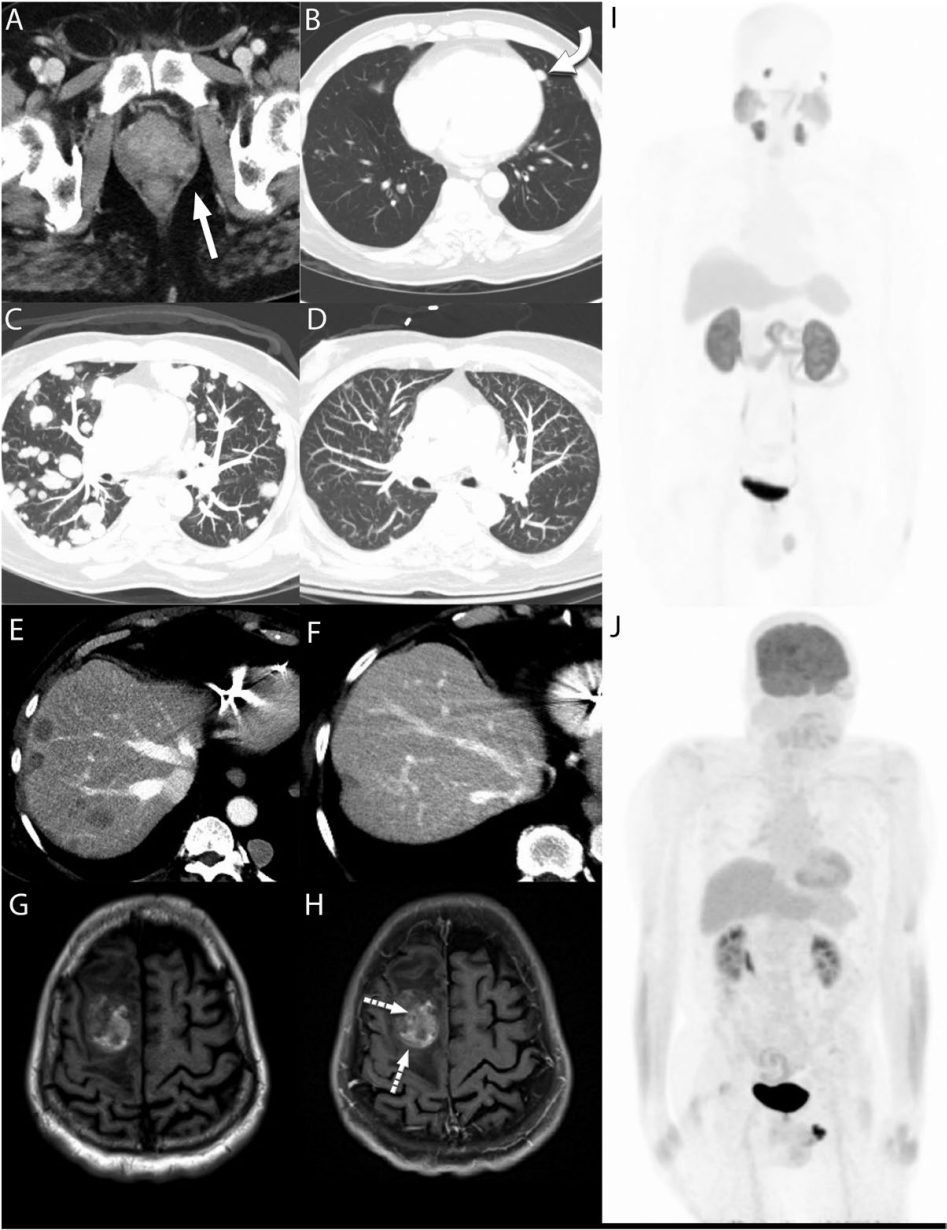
(A) Baseline axial CT image showing heterogenous enhancement of the prostate. (B) Axial CT image demonstrating a dominant 10 mm lingular nodule suspicious for metastatic disease. (C) Axial chest CT image 2 months after baseline demonstrating marked progression of pulmonary metastatic nodules. (D) Axial chest CT image 10 months after baseline with near resolution of pulmonary nodules with some residual opacities likely representing post treatment change. (E) Axial CT image demonstrating multiple progressive liver metastasis 2 months after baseline CT. (F) Axial CT image demonstrating resolution of liver metastasis 10 months after baseline. (G) Pre-contrast axial T1 image demonstrating a hemorrhagic mass in the right frontal lobe with associated mass effect. (H) Post-contrast axial T1 image demonstrating subtle enhancement of the mass that was suspicious for metastasis. (I) MIP image from Pylarify PET/CT with no appreciable abnormal uptake in the lungs or liver 7 months after diagnosis. (J) MIP image from FDG PET/CT 8 months after diagnosis demonstrating no appreciable suspicious uptake. Lung nodules were decreased in size and demonstrated low level non-specific uptake.
